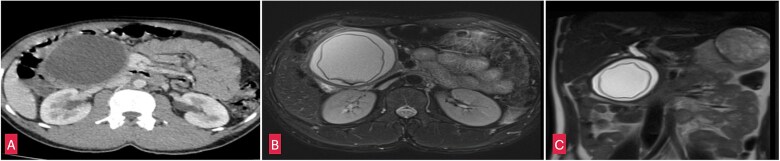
(A) Coronal section of CECT abdomen showing a thick walled, well defined round to oval cystic lesion ~7.5 × 8 × 10 cm in the head and uncinate process of pancreas. (B) Coronal section of MRI-magnetic retrograde cholangio-pancreatography (MRCP) abdomen showing a well defined thick walled cystic lesion in the head and uncinate process of the pancreas with undulating membrane noted within the cyst. (C) Saggital section of MRI-MRCP abdomen with similar findings as above.
